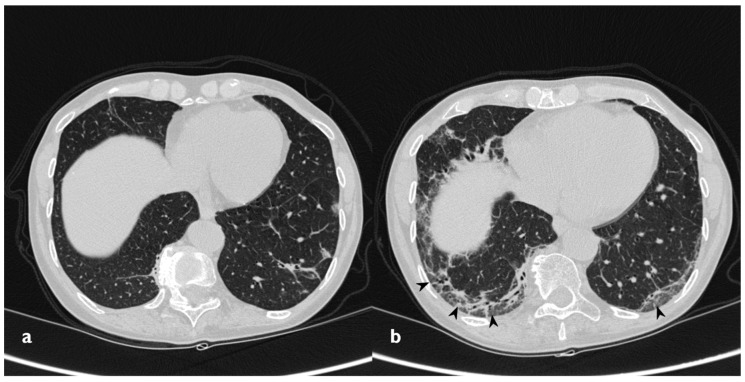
Fibrosing evolution. On the left (a): baseline HRCT. On the right (b): HRCT after 11 months of steroid therapy; new signs of fibrosis-sub-pleural basal reticulations and architectural distortion (arrowheads).
